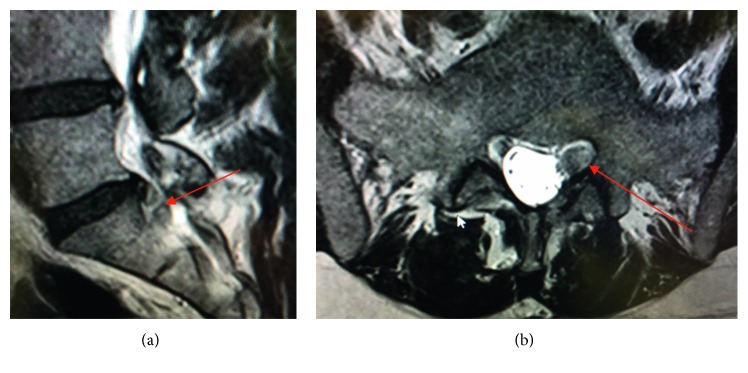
Lumbar MRI of case 3 with left-side S1 compression.
